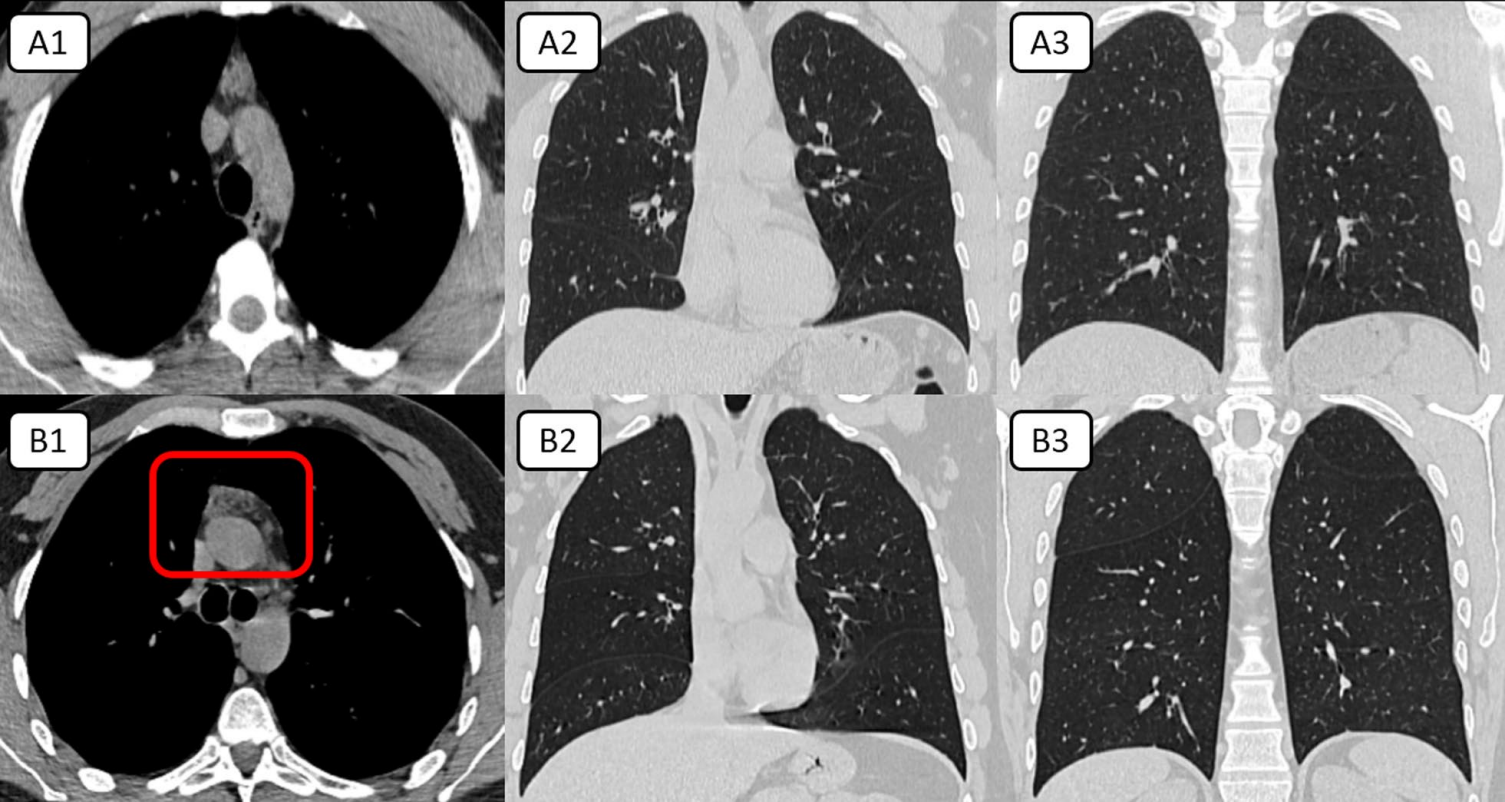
Two COVID-19 patients proved with PCR test results without lung involvement: (A) 31-year-old male patient complained from fever and cough for two days; [A1]: Axial mediastinal window CT showing solid soft tissue replacement of the thymic gland (grade 0). [A2-3]: Axial lung window CT cuts at the level of the cardiac silhouette and para-vertebral planes show clear bilateral lung parenchyma. (B) A 33-year-old male patient complained of fever, cough, and chest pain for three days; [B1]: Axial mediastinal window CT showing a nodular pattern of the thymic gland (grade 2). [B2-3]: Axial lung window CT cuts at the level of the cardiac silhouette and para-vertebral planes show clear bilateral lung parenchyma
